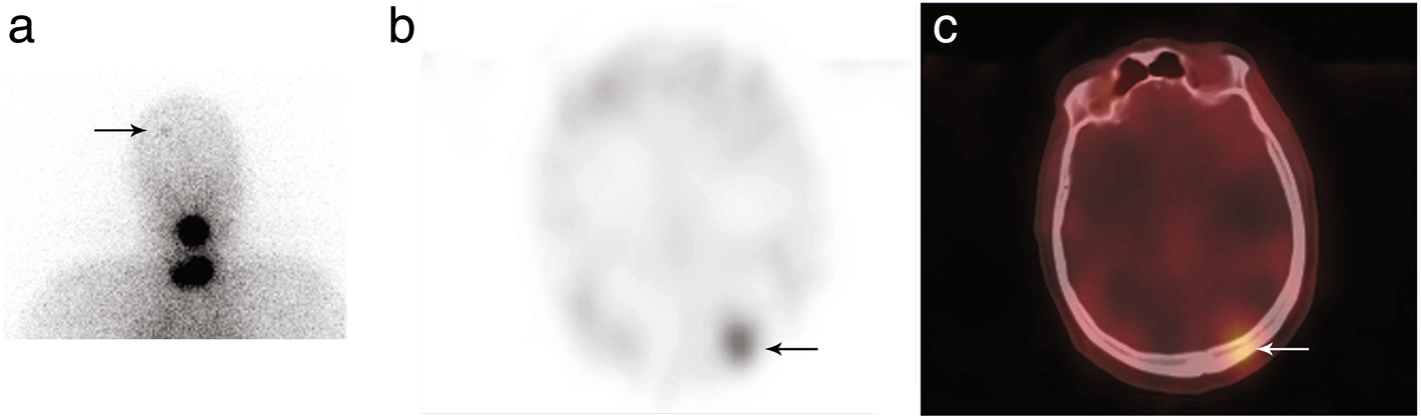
A 56-year-old male patient complained of back pain related to a lytic bone metastasis which led to the discovery of a follicular thyroid cancer. Post-ablation scintigraphy in March 2012 evidenced an intense RAI uptake in front of the spinal metastasis and other uptake in a rib and in the skull. Planar acquisition (posterior view, Panel a), SPECT (Panel b) and fused (Panel c) images of SPECT/CT acquisition displaying the faint RAI uptake in the left part of the skull are consistent with bone metastasis
